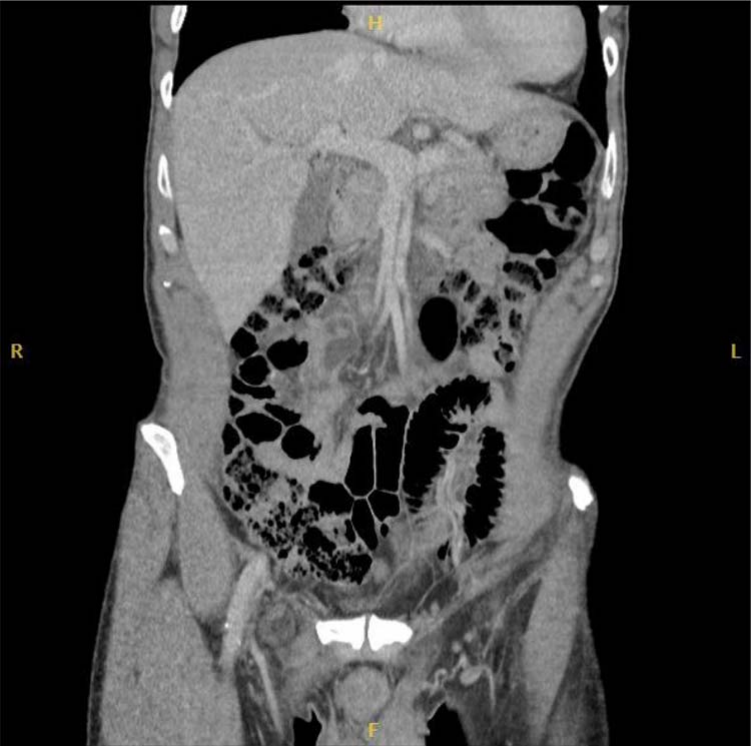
Coronal CT image of right De Garengeot hernia.
